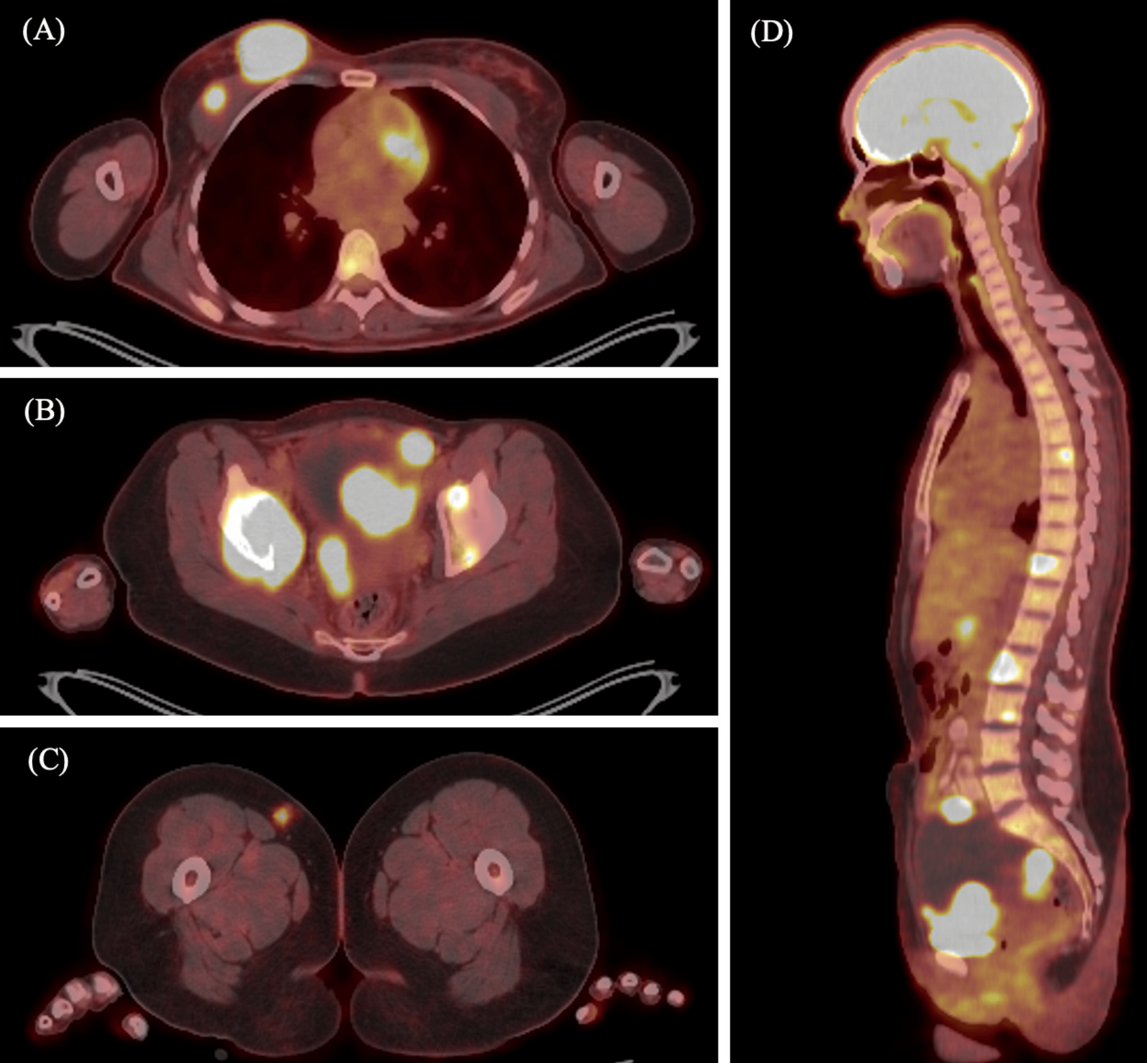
Staging FDG-PET/CT A. Thoracic axial view: FDG-avid right breast lesion and adjacent uptake in the ipsilateral pectoralis major muscle, consistent with soft tissue involvement by extramedullary plasmacytoma. B. Pelvic axial view: FDG uptake in both acetabula and femoral heads, as well as in pelvic lymph nodes and the bladder wall, indicative of widespread extramedullary disease. C. Lower limb axial view: Focal hypermetabolic subcutaneous lesion in the right thigh, suggestive of superficial soft tissue involvement. D. Sagittal view: Diffuse osseous involvement with intense FDG uptake in the thoracic and lumbar vertebrae. Additional hypermetabolic foci are noted in abdominal and pelvic soft tissues. PET/CT: positron emission tomography/computed tomography; FDG: fluorodeoxyglucose
